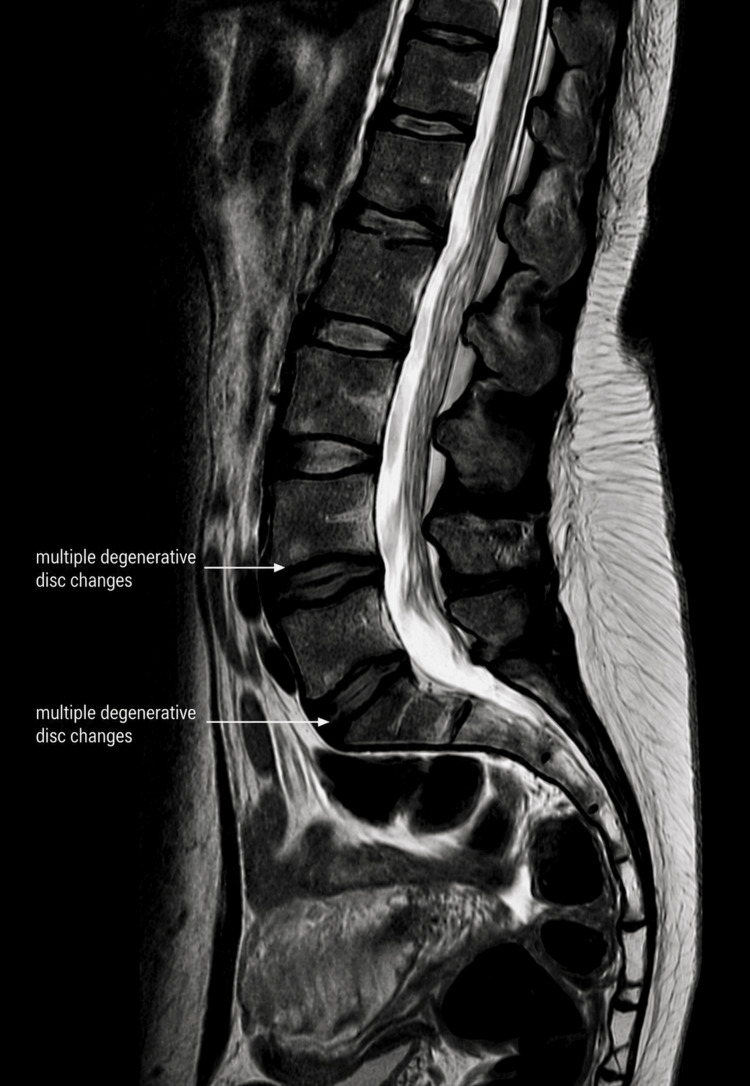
MRI of the lumbar spine MRI of the lumbar spine demonstrating mild degenerative disc changes without a compressive lesion or structural abnormality capable of explaining the recurrent neurological and autonomic manifestations.
